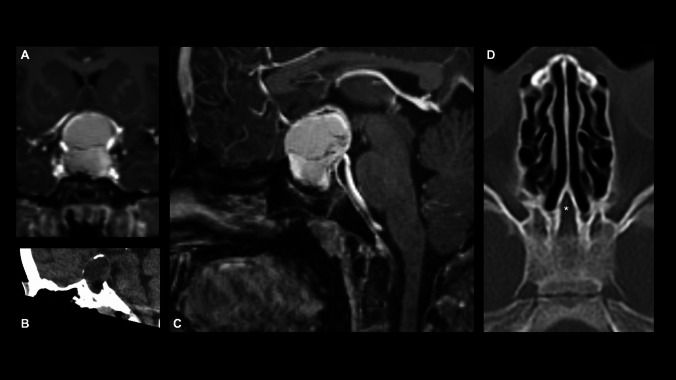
A Coronal T1-weighted magnetic resonance imaging (MRI). B Sagittal soft tissue-weighted computed tomography (CT). C Sagittal T1-weighted MRI demonstrating a 3 × 3 × 2.5 cm cystic space-occupying partially calcified lesion in the sellar and suprasellar compartment with compression of the optic chiasm, highly susceptive of a craniopharyngioma (CP). D Axial bone-weighted CT demonstrating a non-pneumatized sphenoid bone with a hypo intense bone-marrow (*asterisk*)
